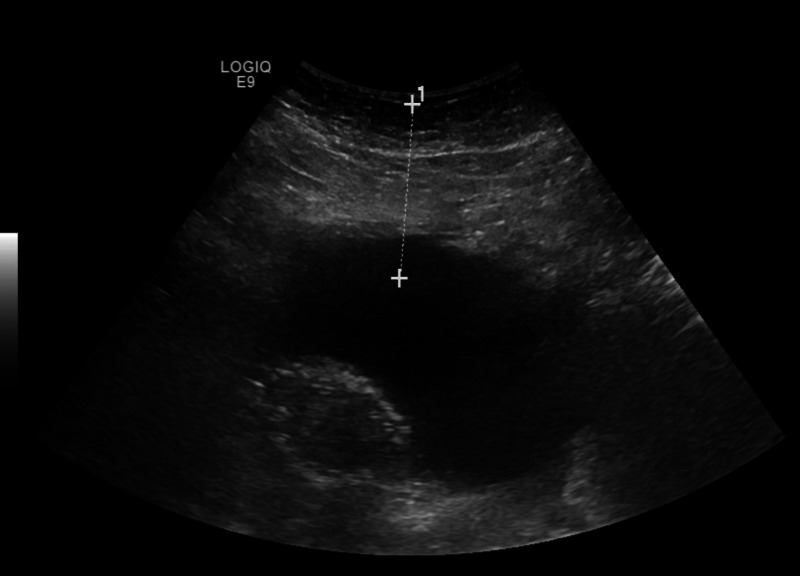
Ultrasonogram (US) abdomen showing free fluid collection in right lower quadrant which was later confirmed to be biliary
